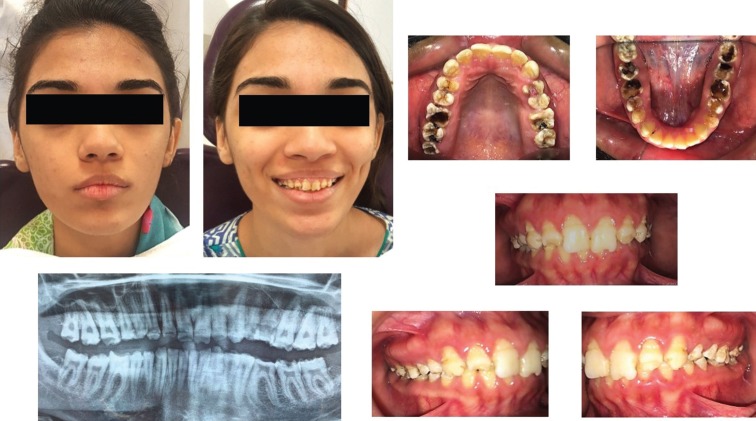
Pre-operative clinical pictures and panoramic radiograph.
